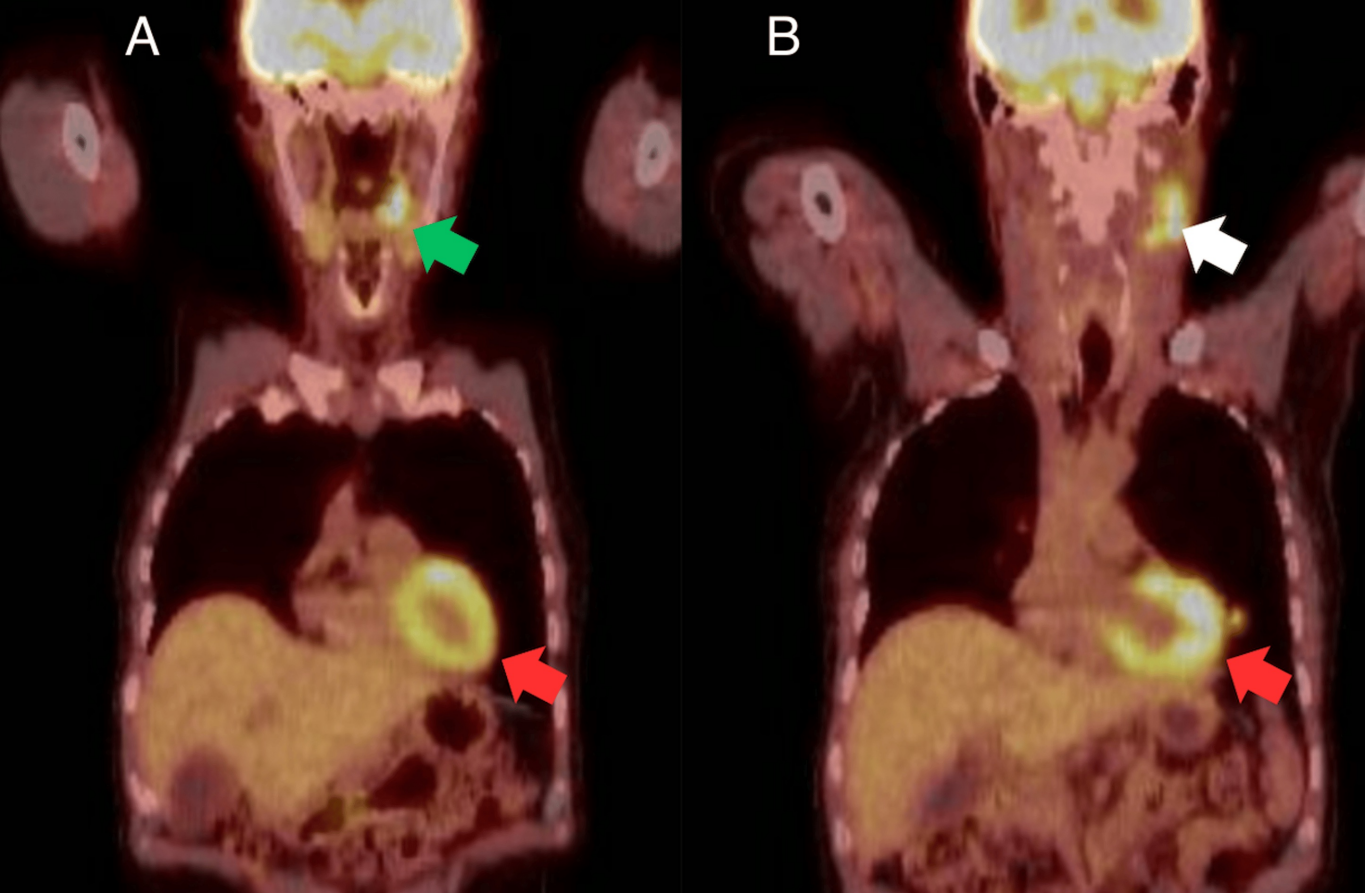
PET/CT demonstrating left peritonsillar, left upper jugular lymph node chain, and left lingula nodule hyperactivity. Image A demonstrates left peritonsillar (green arrow) and left lingula (red arrow) hyperactivity. Image B demonstrates the left upper jugular lymph node chain (white arrow) and left lingula (red arrow) hyperactivity.
